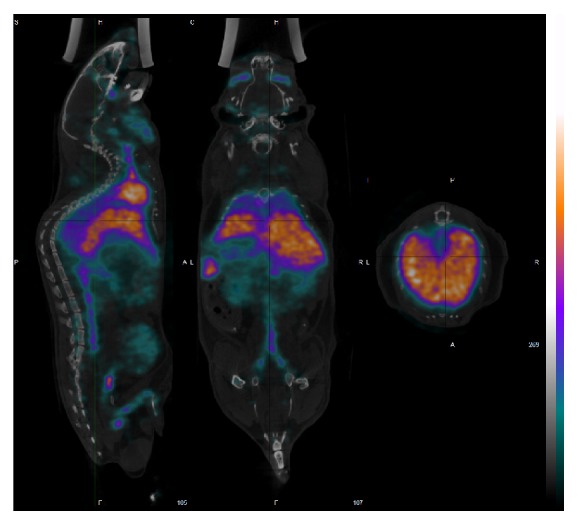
In vivo biodistribution of liposomes labeled with 99m-Technetium. Single photon emission computed tomography combined X-ray computed tomography (SPECT-CT) data were recorded after 1.5 hours of the administration of labeled liposomes. The distribution reflects that of non-PEGylated liposomes and shows high uptake by the liver.
